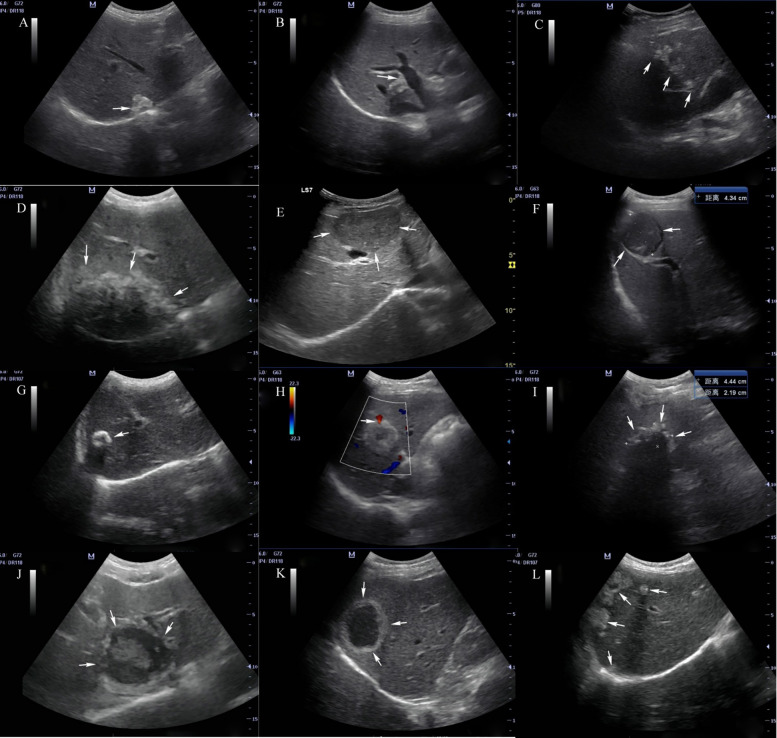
Ultrasonographic manifestations of hepatic alveolar echinococcosis Arrows in the figure: (A) hyperechoic nodular lesion; (B) angulation deformity of lesion edge; (C) treelike or beaded growth; (D) infiltration type; (E) mass type; (F) tumor-like sign; (G) calcification type; (H) vascular truncation sign; (I) “hailstorm” sign; (J) “island” sign; (K) “pseudocyst” type; (L) multiple AE lesions in liver.
